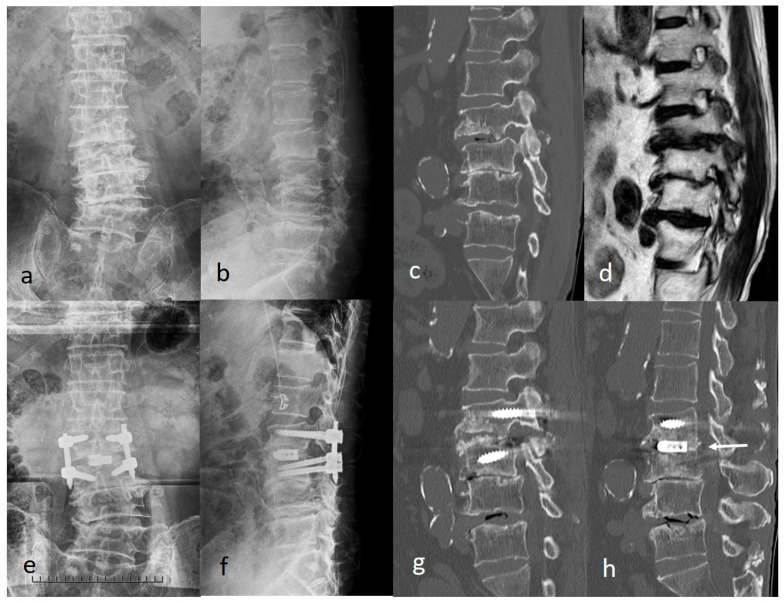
Case of left-sided foraminal stenosis at the L2/3. (a,b) Preoperative radiograph. (c,d) Preoperative computed tomography and magnetic resonance imaging revealing left-sided foraminal stenosis at L2/3. (e,f) Postoperative radiograph. (g) Postoperative computed tomography revealed a significant reduction in foraminal stenosis. (h) Intervertebral bone fusion posterior to the cage (white arrow).
